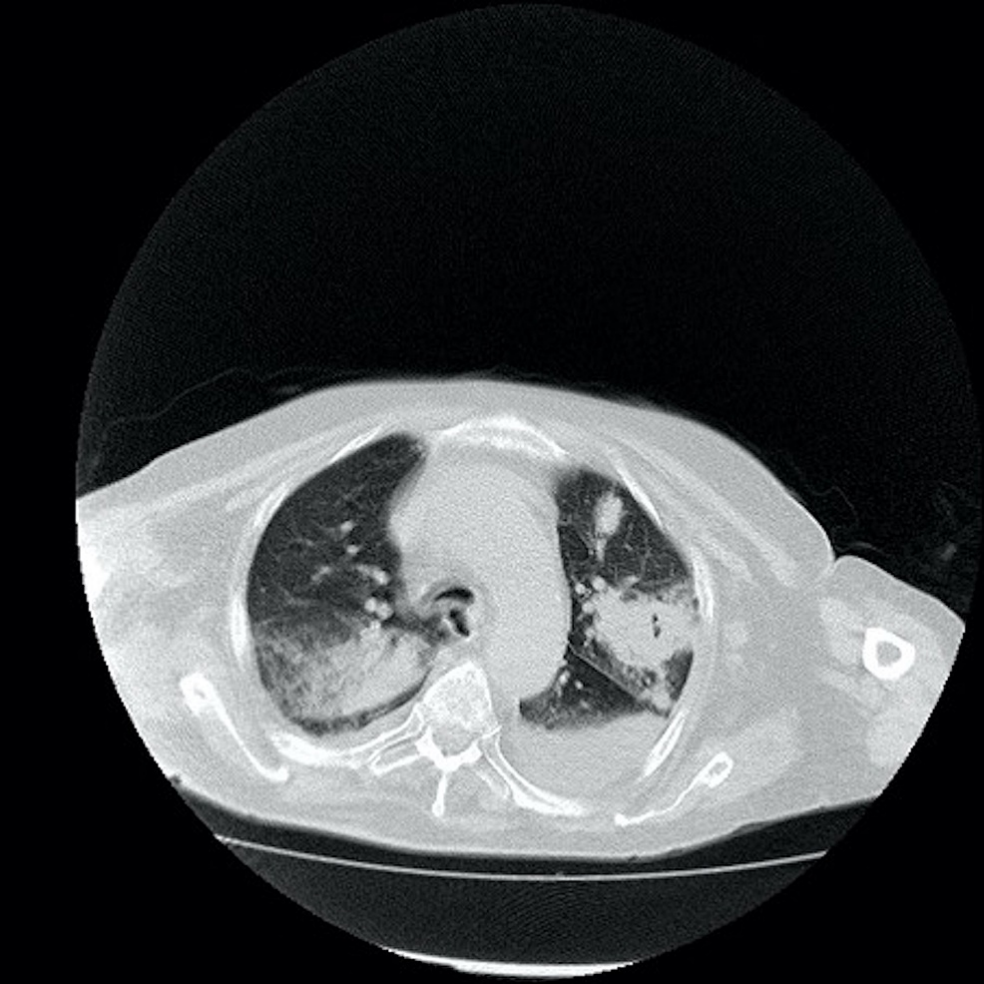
Bilateral patchy ground-glass opacities and consolidation were observed in thorax computed tomography.
